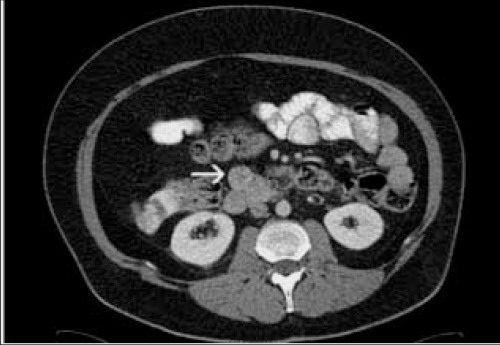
CT shows a nodular lesion approximately 2 cm in diameter(arrow)
